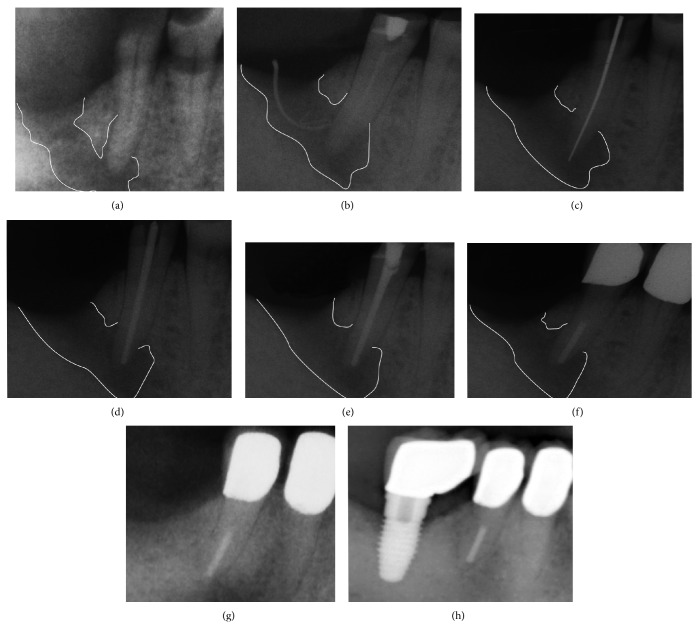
(a) Periapical radiograph prior to root canal treatment. (b) Tracking of the sinus tract with gutta-percha point. (c) Determination of working length. (d) Master point radiograph. (e) Completed root canal treatment. (f) Reconstruction with fibre post and metal-ceramic crown; partial healing of the bone defect. (g) Radiographic situation after complete healing. (h) Radiographic situation of the implant after five years in situ.
